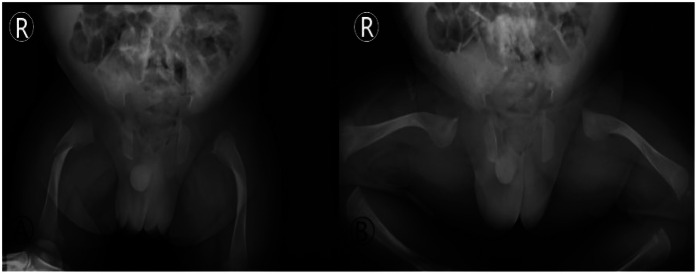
Imaging of the first spontaneous fracture post-discharge (day 25 of life). Anteroposterior (AP) and frog-leg lateral radiographs of the hips: (A) (AP view): a fracture line with slight angulation is visible in the proximal right femur (red arrow). (B) (FroG–Leg lateral view): the inherent bony abnormalities of OI, including bowing and thin cortices, are also seen. This image documents the first “traumatic” event during the home care phase, highlighting the high vulnerability during the post-discharge transition.
